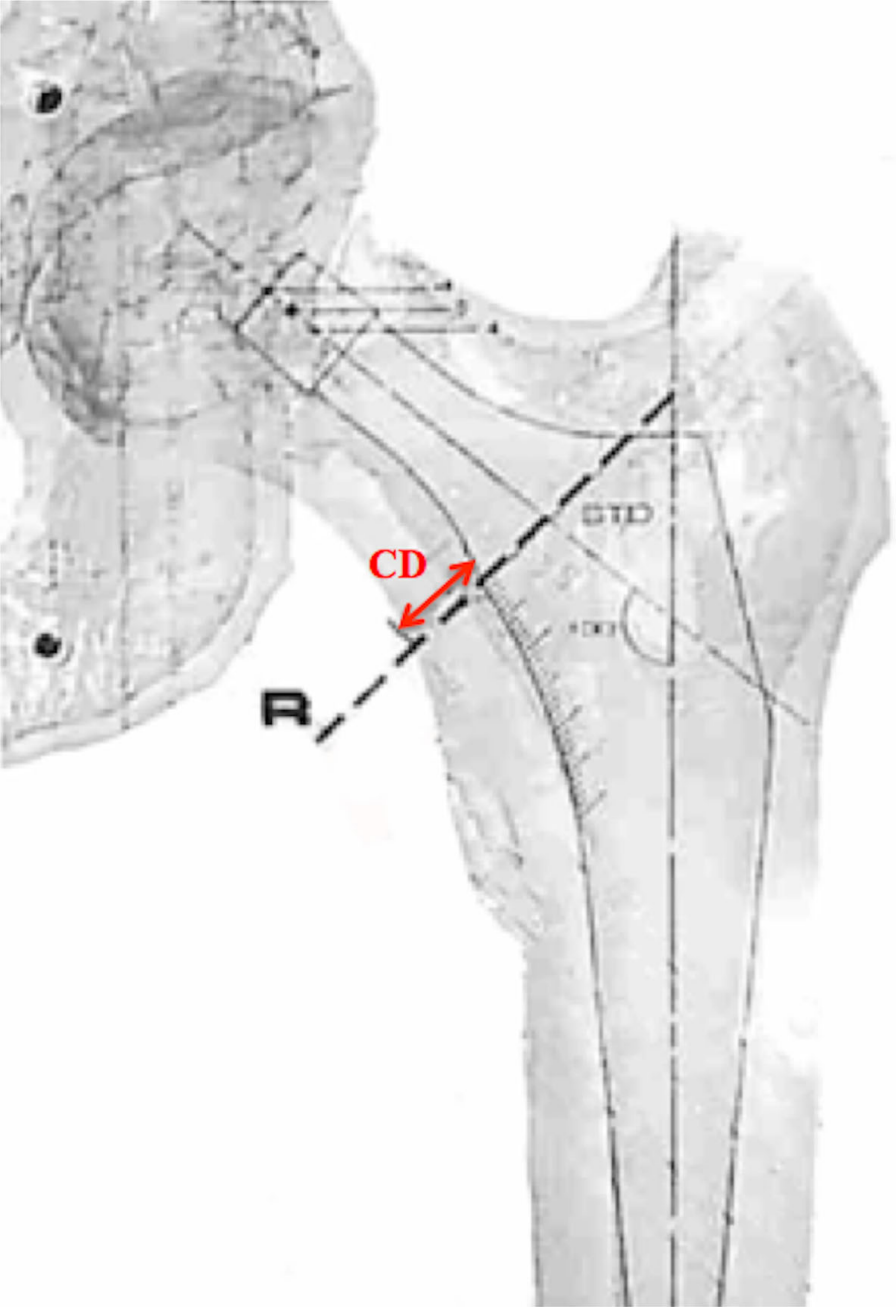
Templating femoral stems on printed CT-scans to select the size and model of the stem matched and to measure the cortical distance (CD) at the level of the collar resection (femoral calcar)
